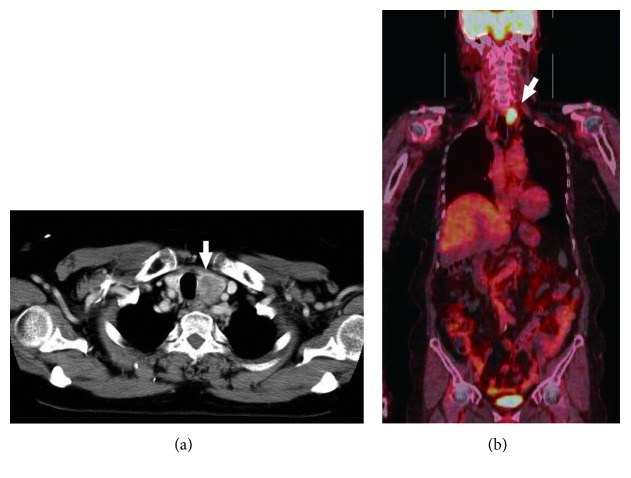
Enhanced CT and PET-CT before surgery. CT demonstrates spread of the mass to the tracheoesophageal groove, suggesting invasion of the left recurrent laryngeal nerve (a). PET-CT shows focal uptake in the left thyroid lobe, with no evidence of distant metastasis (b). The mass is indicated by arrows. CT, computed tomography; PET, positron emission tomography.
